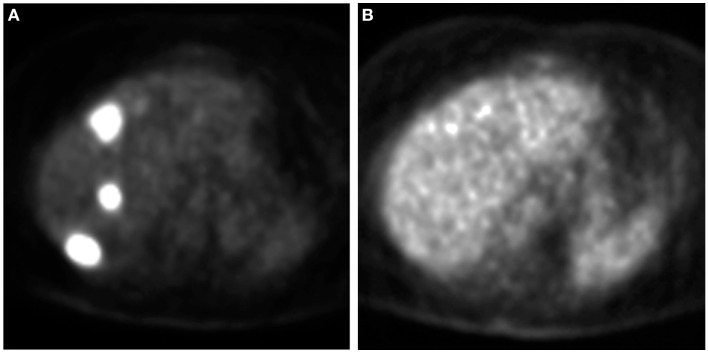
Assessment of response to Y-90 therapy on PET. A PET scan (A) obtained prior to Y-90 therapy demonstrates three FDG avid lesions. A repeat PET scan (B) obtained 6 weeks after Y-90 therapy shows no FDG avid lesions suggesting complete metabolic response.
